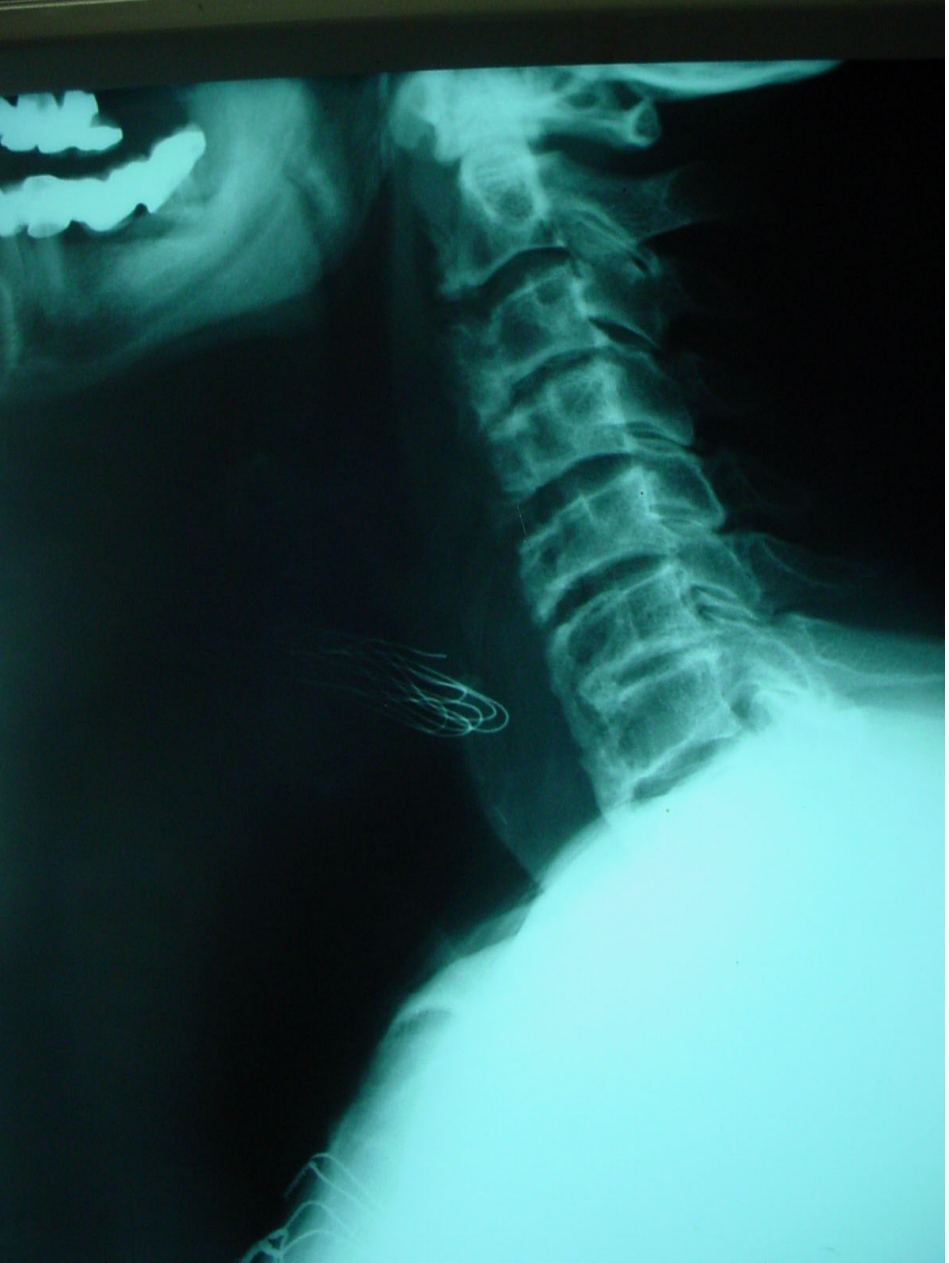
Postoperative lateral cervical spine x-rays showed absence of the osteophytes and normalization of the normal structure.
